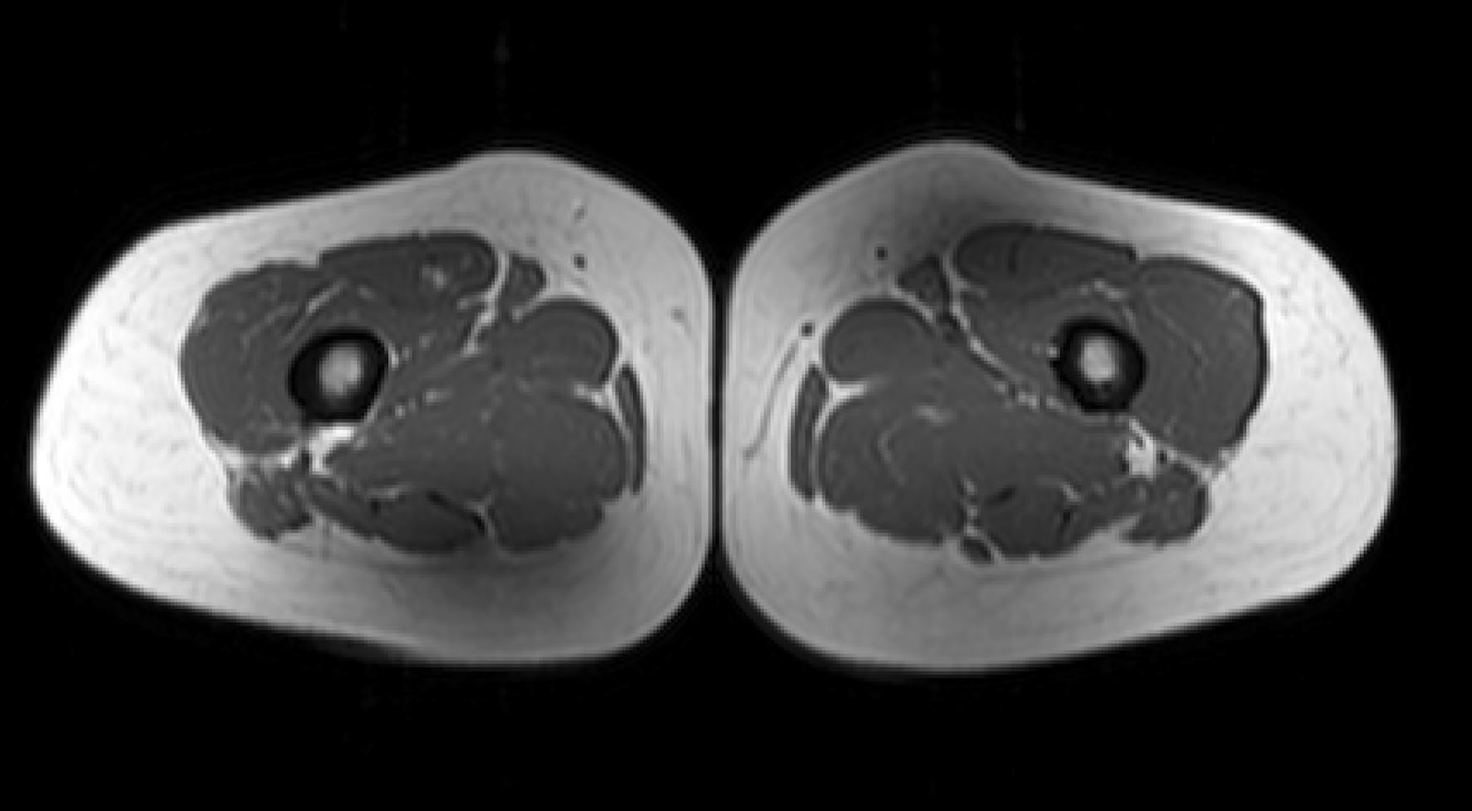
T1-weighted image of dystrophic thigh muscle. The widely varying signal intensity in the uniform subcutaneous fat demonstrates the B_1_ inhomogeneity across the leg at 3.0 T which inhibits the ability of T1-weighted images to monitor disease progression
